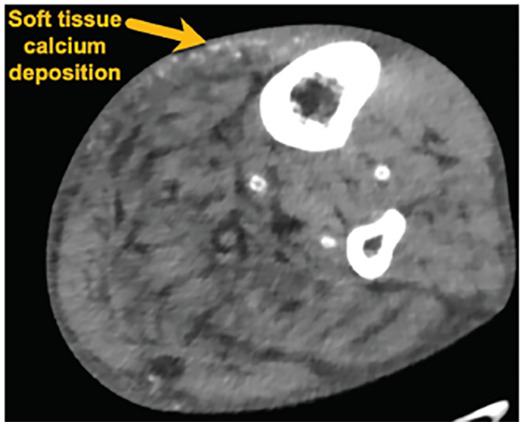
Computed tomography with axial view demonstrating soft tissue calcium deposits diagnostic of calciphylaxis in a patient with end-stage renal disease.
